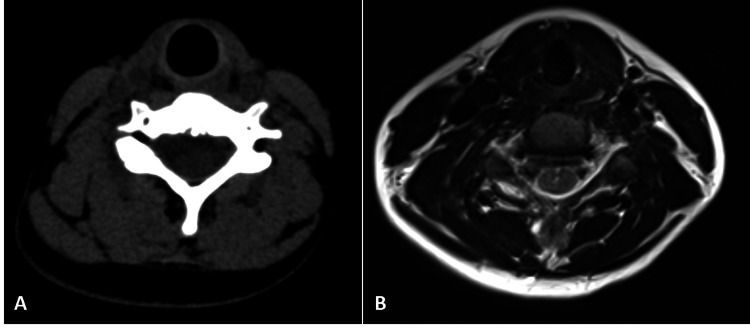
Non-visualisation of the thyroid gland in its normal anatomical position on CT (A) and MRI (B) images.
